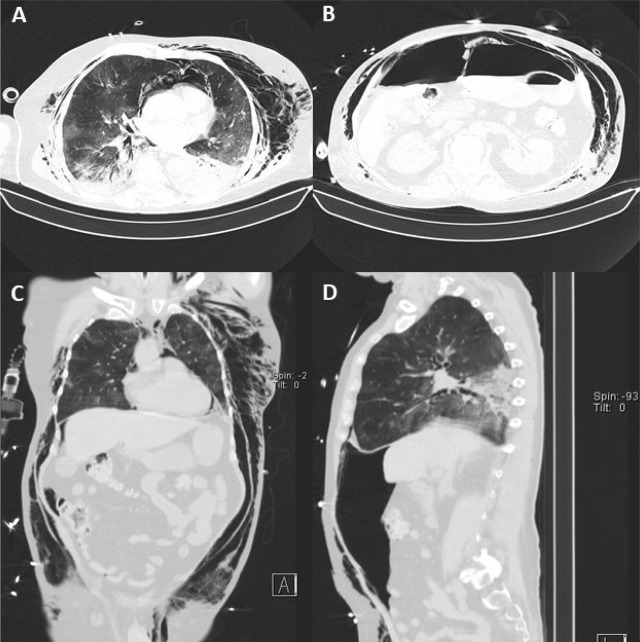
Thoraco-abdominal CT showing SE and moderate pneumomediastinum (axial - A, coronal - C) and a large pneumoperitoneum (axial - B, sagital - D).
